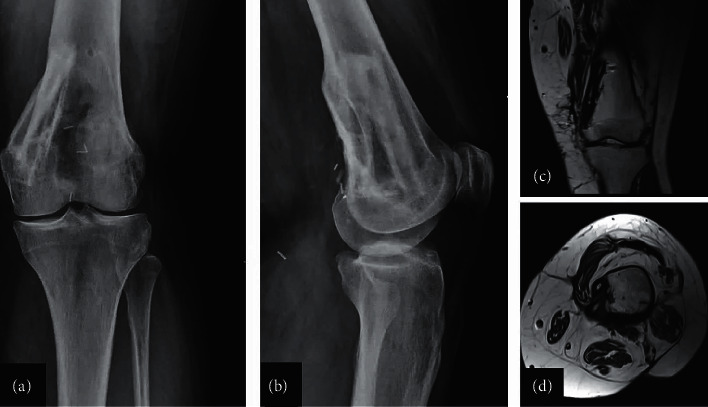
Anteroposterior (a) and lateral (b) radiographs of the knee 13 months postoperatively showing incorporation of the fibula autografts with no signs of recurrence. Magnetic resonance imaging of the distal femur (sagittal (c) and axial (d) views) showing the distal femur with the fibula autografts with no signs of recurrence, infection, or autograft fracture.
